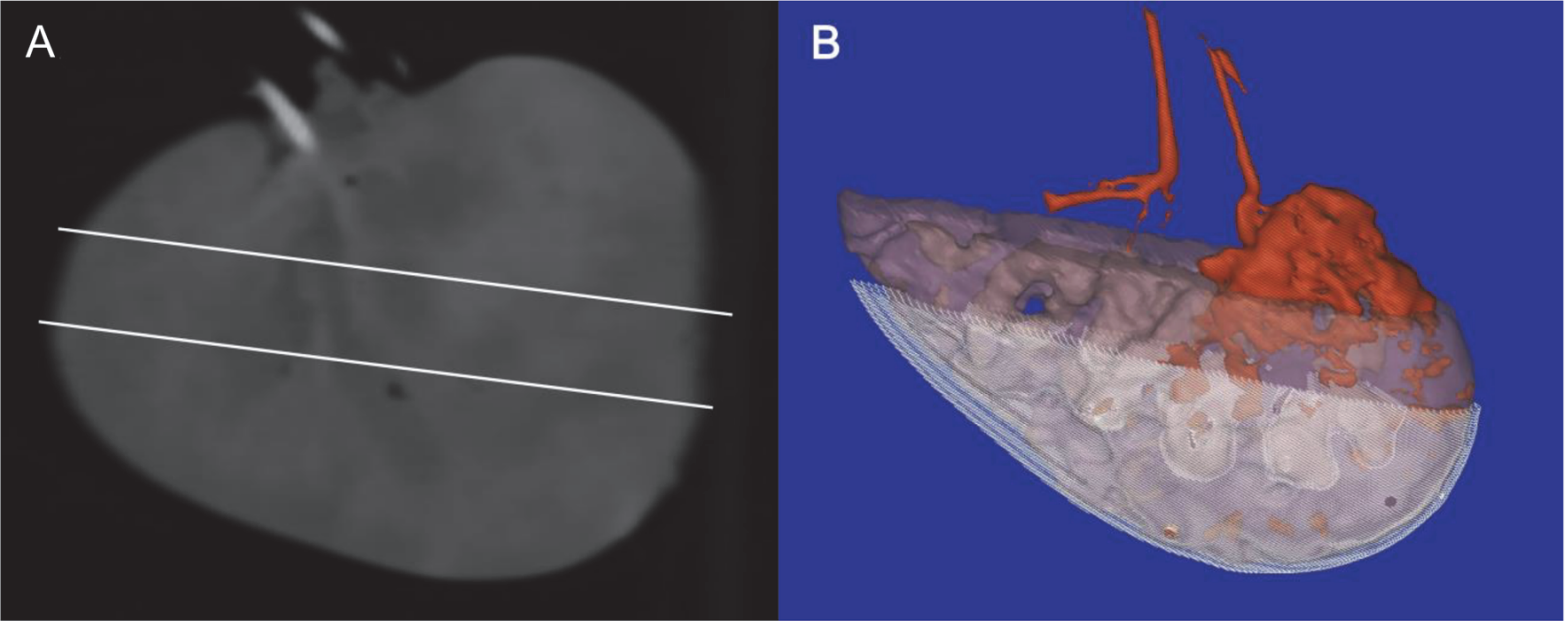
Renal spreading of Thiel embalming fluid. (A) Planar CT image shows three areas of filling (contrast): bright, the main arterial and venous system and the poles-medial border of the kidney; intermediate grey, centrally distributed areas; and darker grey, areas at the core and kidney surface. Two oblique white lines represent virtual slicing through the upper and lower mid-central part of the kidney. (B) Three-dimensional representation of the same kidney; red, main arterial and venous system and one of the poles-medial border areas; transparent purple, centrally distributed areas of the kidney showing intermediate contrast filling; and white polylines, inner core areas (four cone-like structures, calices) and surface of the kidney showing the least contrast filling.
